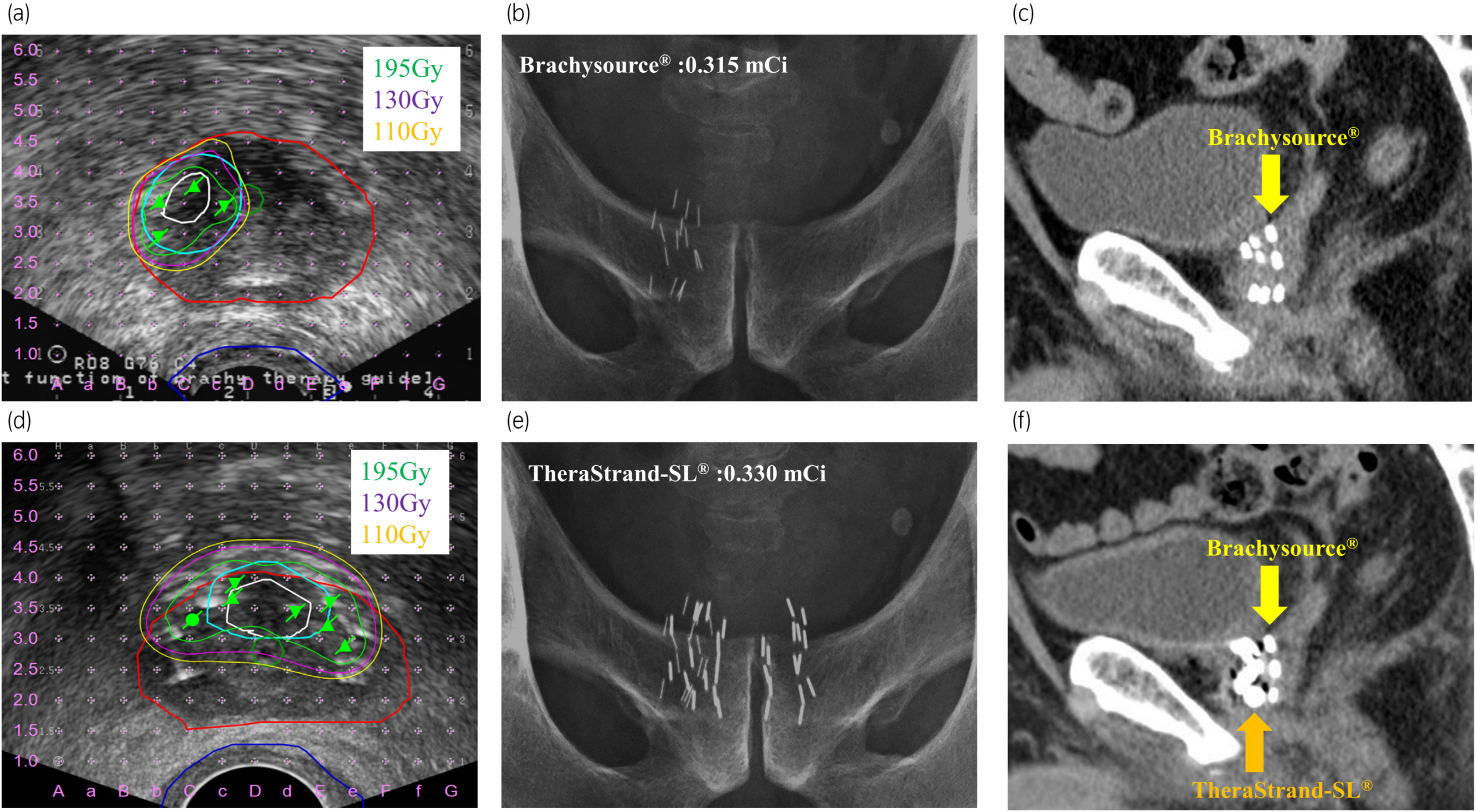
Dose distributions and pelvic X‐rays of the seed implantations. The red, green, blue, light blue, and white lines indicate prostate, urethra, rectum, PTV, and tumor based on MRI, respectively. (a) Intra‐implant dosimetry of salvage fLDR brachytherapy on transrectal ultrasound. The PTV was defined as the tumor area plus a 3‐mm margin on the anterior, posterior, right, and left sides based on multiparametric 3 Tesla MRI. (b) Pelvic X‐ray of the 15 seeds (Brachysource®) that were implanted in salvage fLDR brachytherapy. (c) Pelvic CT of the seeds (Brachysource®) that were implanted in the salvage fLDR brachytherapy. Brachysource® was displayed as an elongated high‐density area on the CT. (d) Intra‐implant dosimetry of re‐salvage fLDR brachytherapy on transrectal ultrasound. The PTV included the tumor site plus a 3‐mm margin on the anterior, right, and left sides based on multiparametric 3 Tesla MRI, but excluded the posterior margin to reduce the radiation dose to the urethra. (e) Pelvic X‐ray of the 30 seeds (TheraStrand‐SL®) that were implanted in re‐salvage fLDR brachytherapy, in addition to the 15 seeds implanted in salvage fLDR brachytherapy. (f) Pelvic CT of the seeds (TheraStrand‐SL®) that were implanted in the re‐salvage fLDR brachytherapy, in addition to other types of seeds that were implanted in the salvage fLDR brachytherapy. TheraStrand‐SL® was displayed as a thicker and rounder high‐density area than Brachysource® on the CT.
